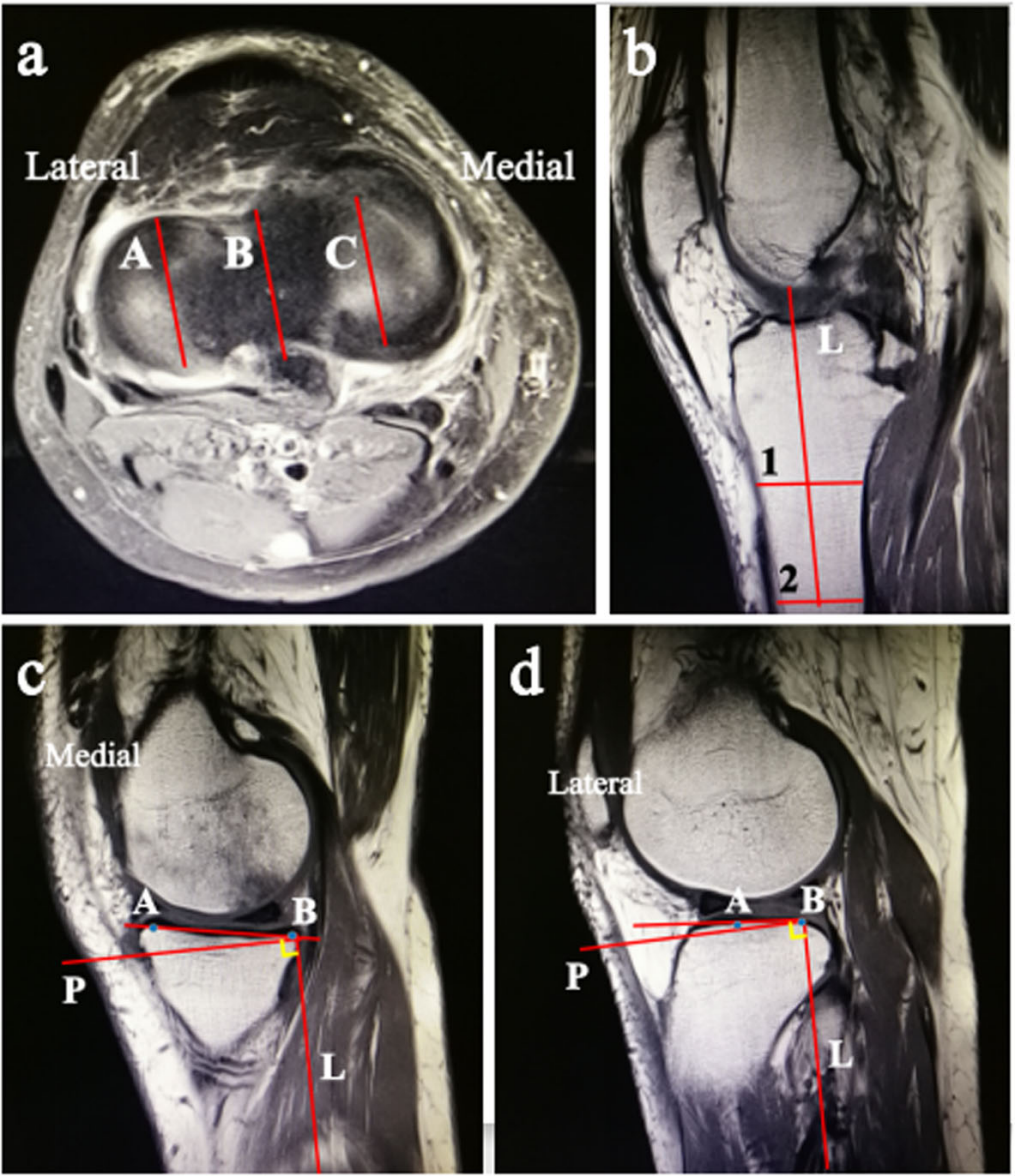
Magnetic resonance images depicting the method used to obtain the sagittal slopes of the medial and lateral tibial plateaus. a This shows the transverse plane passing through the tibiofemoral joint and showing the dorsal aspect of the tibial plateau. Lines A and C show the corresponding locations of the sagittal planes in the medial and lateral tibial plateaus. Line B in the same figure shows the approximate location of the sagittal plane that was used to determine the orientation of the longitudinal axis. b The longitudinal axis (line L) of the tibia was obtained by determining the midpoint of the anterior and posterior widths of the tibia at two points located as distally in the image as possible (locations 1 and 2). c The medial tibial slope was the angle between a line joining the peak points on the medial plateau (points A and B) and line P perpendicular to line L. d The lateral tibial slope was the angle between a line joining the peak points on the lateral plateau (points A and B) and line P perpendicular to line L
